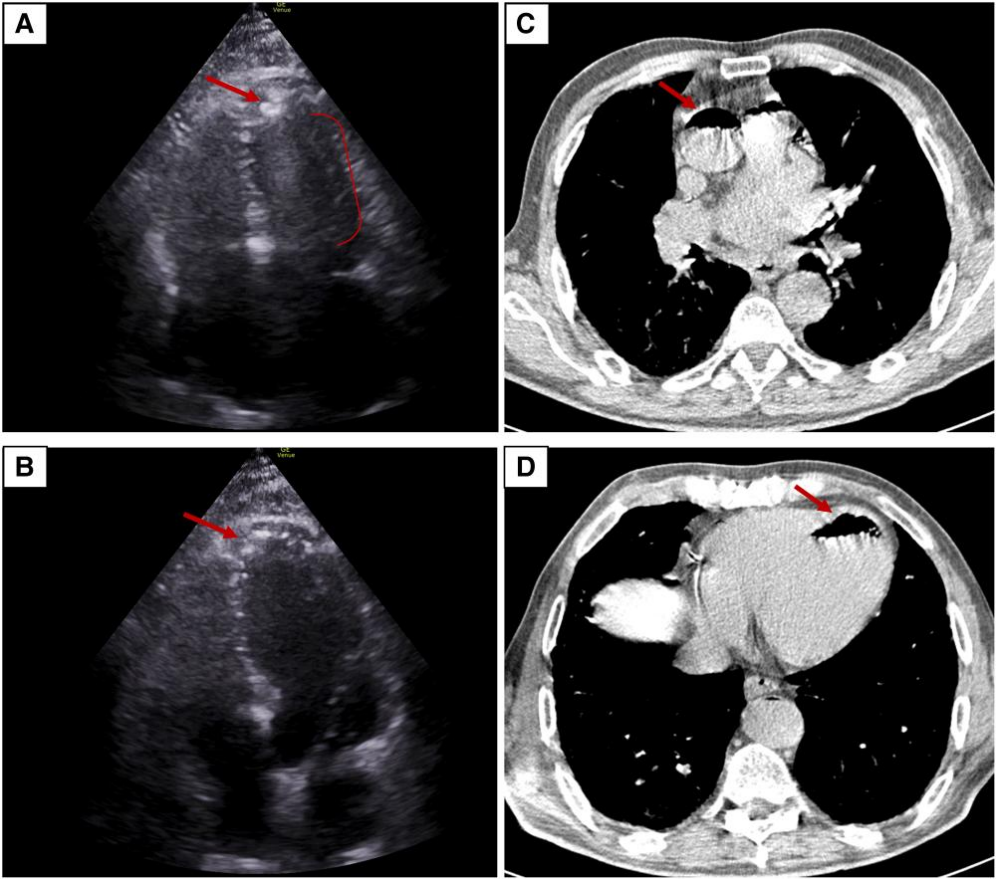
Systemic arterial air embolism following percutaneous lung biopsy. Following sudden-onset coughing and cardiac arrest during lung biopsy, transthoracic echocardiography (*A, B*) revealed air bubbles in the left ventricle (arrow), associated with a dynamic, cone-shaped artefact synchronous with the cardiac cycle. Chest computed tomography confirmed the presence of air (arrows) within the ascending aorta (*C*) and left ventricle (*D*).
